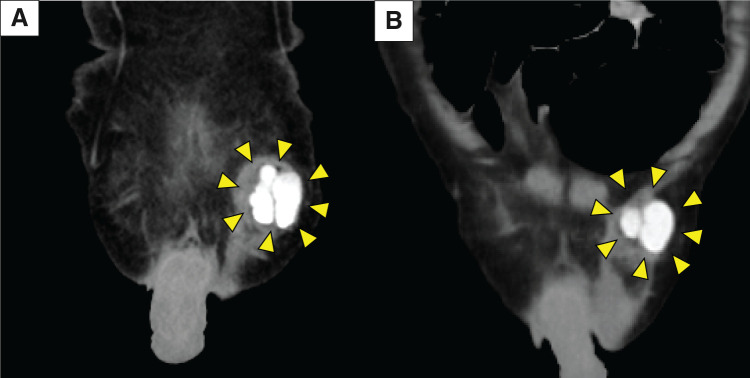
CT findings. (A) A lobulated, hyperdense lesion (maximum diameter, 31 mm; attenuation, 196 HU) is present in the left inguinal canal (arrowheads). (B) One year earlier, a similar lesion measuring 26 mm had been detected (arrowheads).
